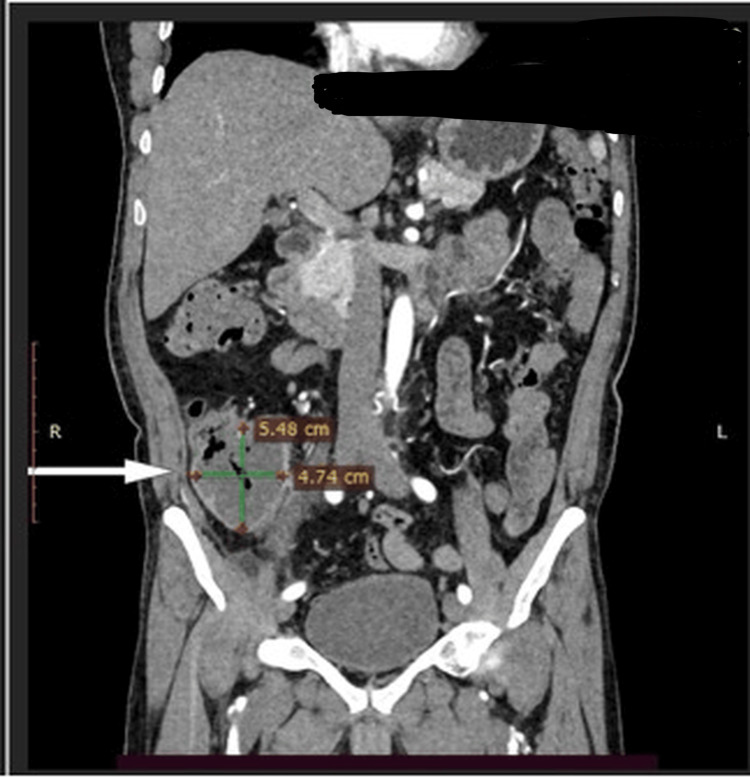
Contrast-enhanced CT abdomen suggestive of a necrotic collection of approximately 40 to 50 cc at the base of appendix
